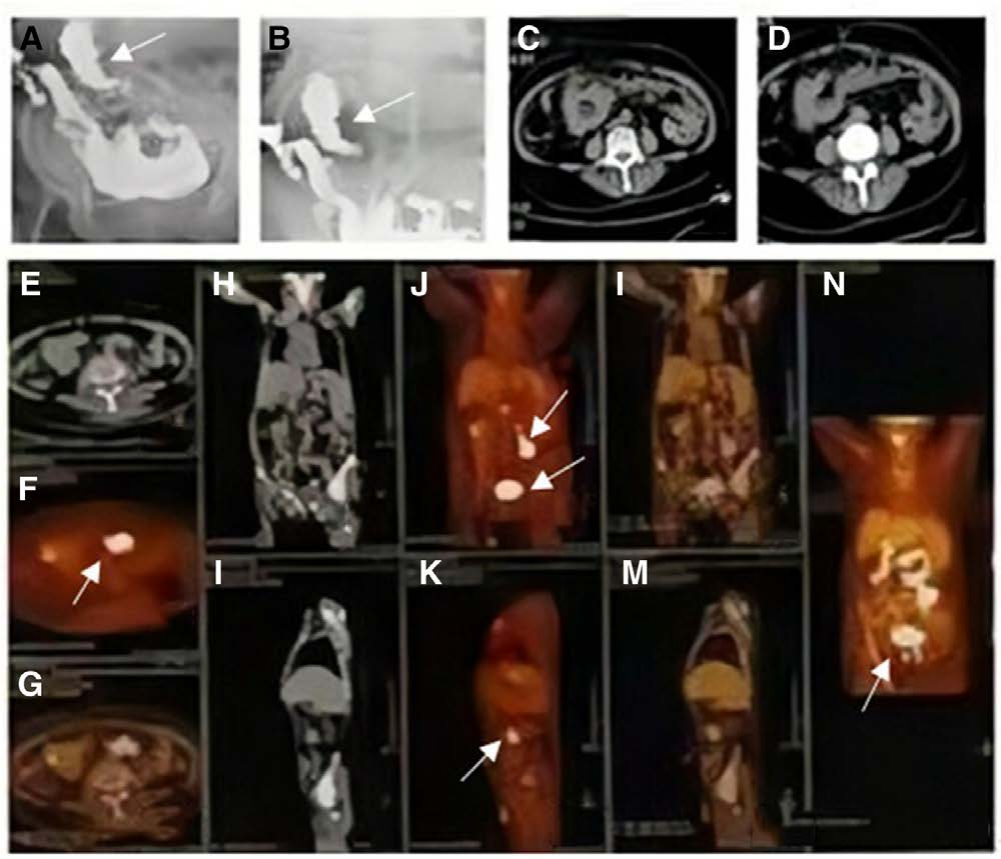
The arrow in (A and B) showed local small intestinal lesions in the right mid-abdomen and abdominal enhanced CT (C and D) of the patient. The arrow in (E–N) showed Abnormal glucose metabolism was observed in the upper abdomen and pelvic small intestine.
